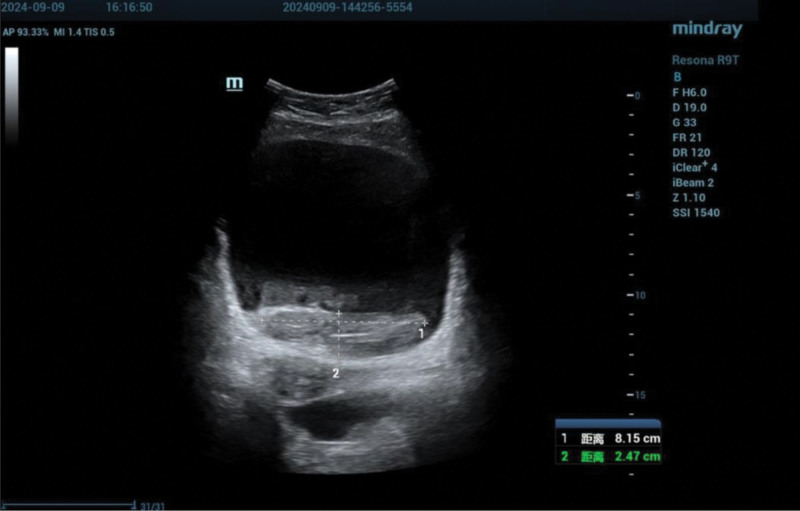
Ultrasonography revealing an 8.15 × 2.47 cm intravesical hematoma. AP = anteroposterior, DR = dynamic range, FR = frame rate, MI = mechanical index, SSI = spatial standard intensity, TIS = thermal index scan.
